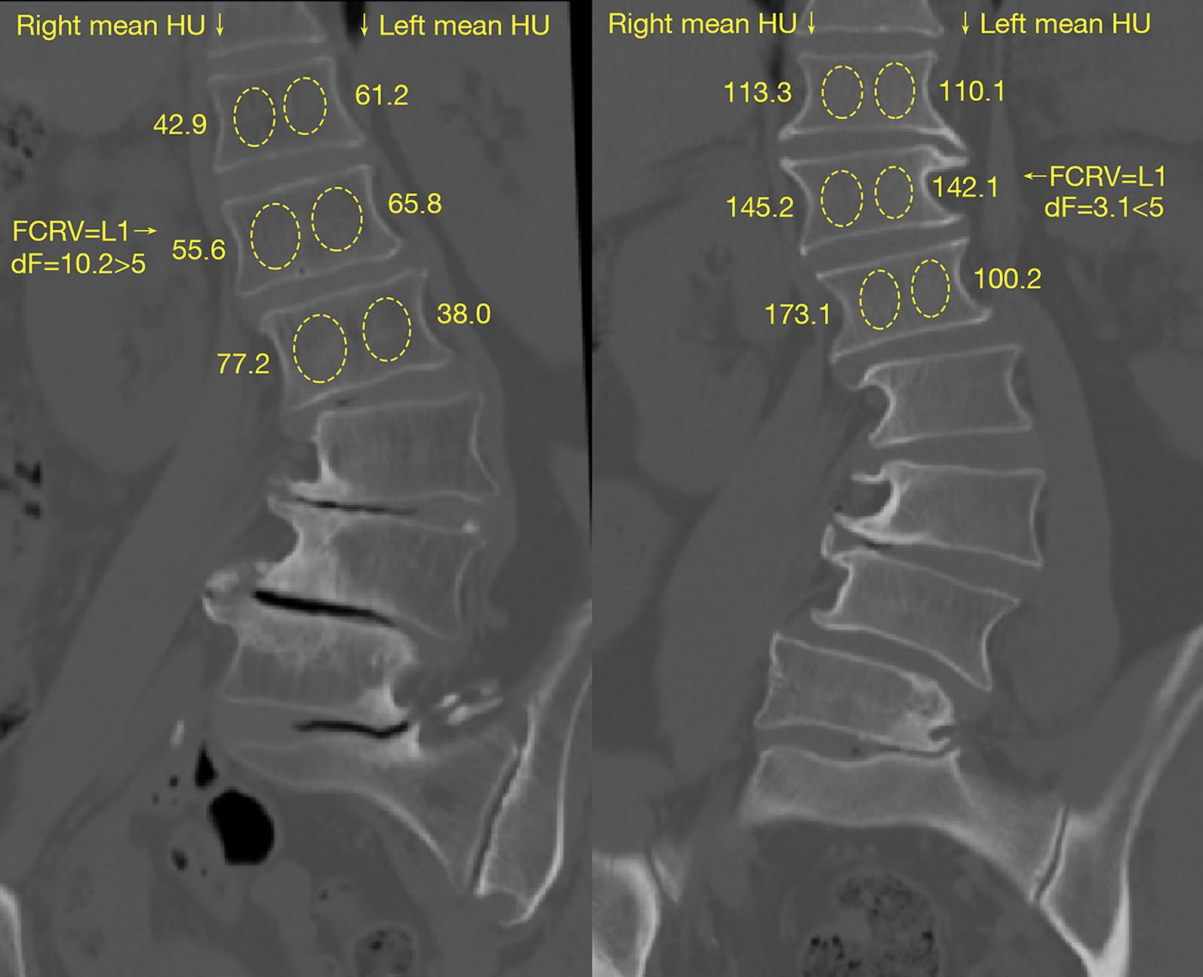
The coronal reconstruction images of the preoperative lumbar CT scans of a dF > 5 patient (a) and a dF < 5 patient (b). The circles show the ROIs measuring HU on one of the 3 slices, while the numbers beside them show the mean HU from the 3 slices. The FCRV marks the turning point of HU asymmetry
